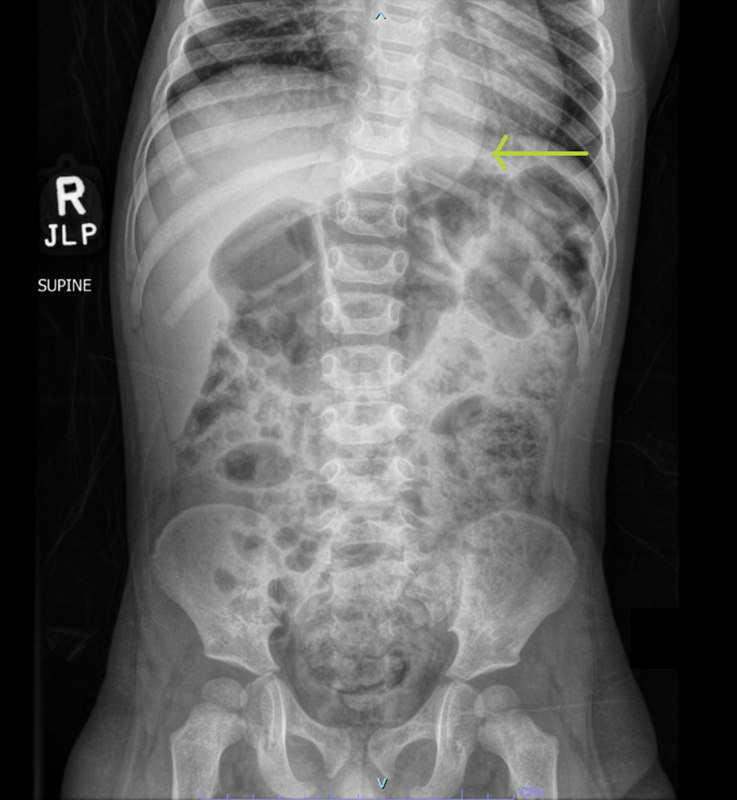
A supine abdominal X-ray upon presentation incidentally demonstrates a smooth, circular paraspinal mass at the level of the diaphragm (arrow).
